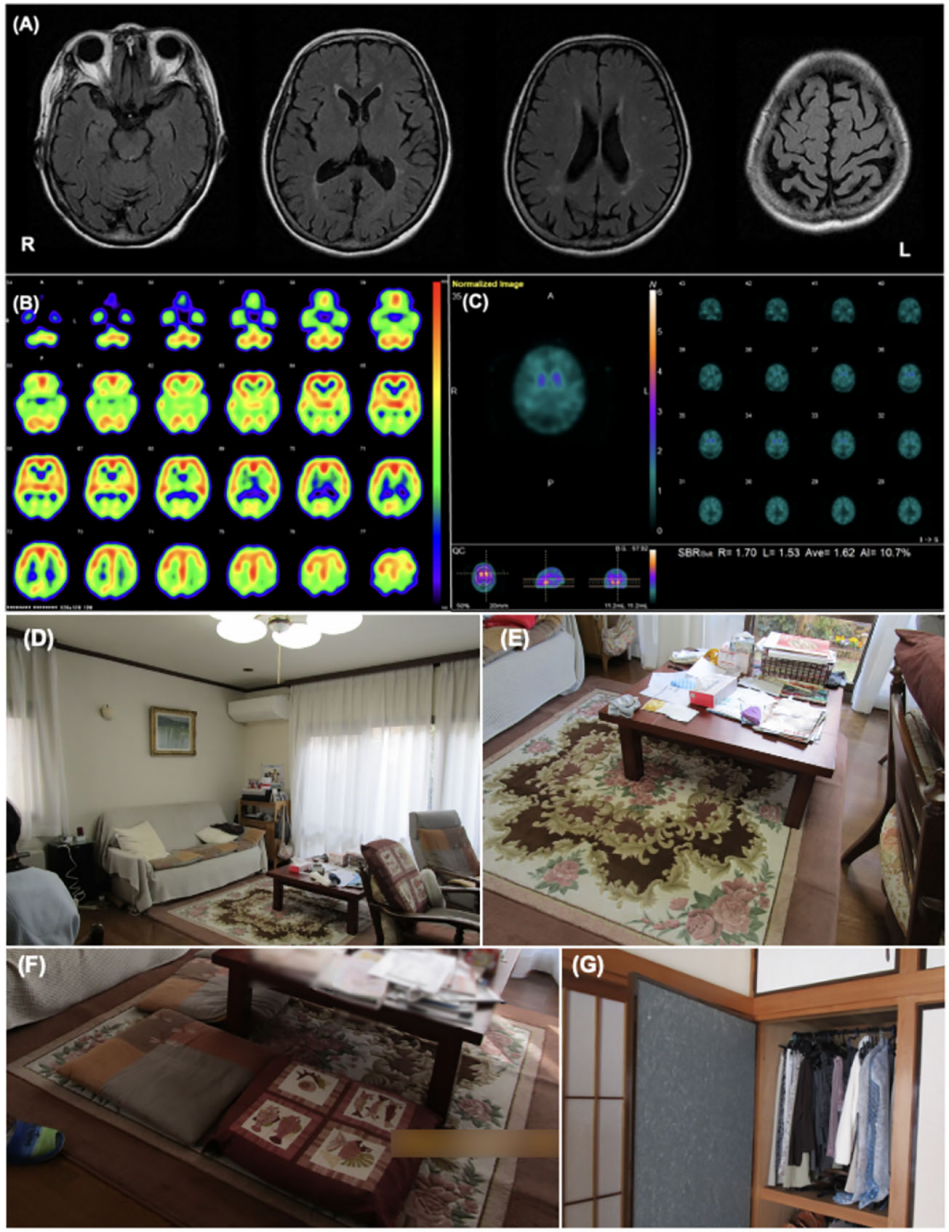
Patient 1 images (A) The cranial MR T2 FLAIR image shows no obvious abnormal findings except for mild atrophy of the bilateral parietal lobes. (B) I-^123^IMP SPECT shows reduced cerebral perfusion in the bilateral parietal, temporal, and occipital lobe while perfusion in the bilateral posterior cingulate cortex was relatively preserved. (C) I-^123^FP-CIT SPECT showed a decrease in accumulation of dopamine in the striatum. (D, E) These images show the environmental triggers associated with the visual hallucinations. Cushions on a sofa in the living room (D), the pattern on a carpet under the table in the living room (E) were suspected of inducing her visual hallucinations as follows: “Boy sitting on the sofa” and “Child playing under the table,” respectively. (F, G) These images show the living environment post-intervention. The carpet pattern was hidden by a tablecloth (F), and the clothes on hangers in the bedroom were stored in a closet (G).
